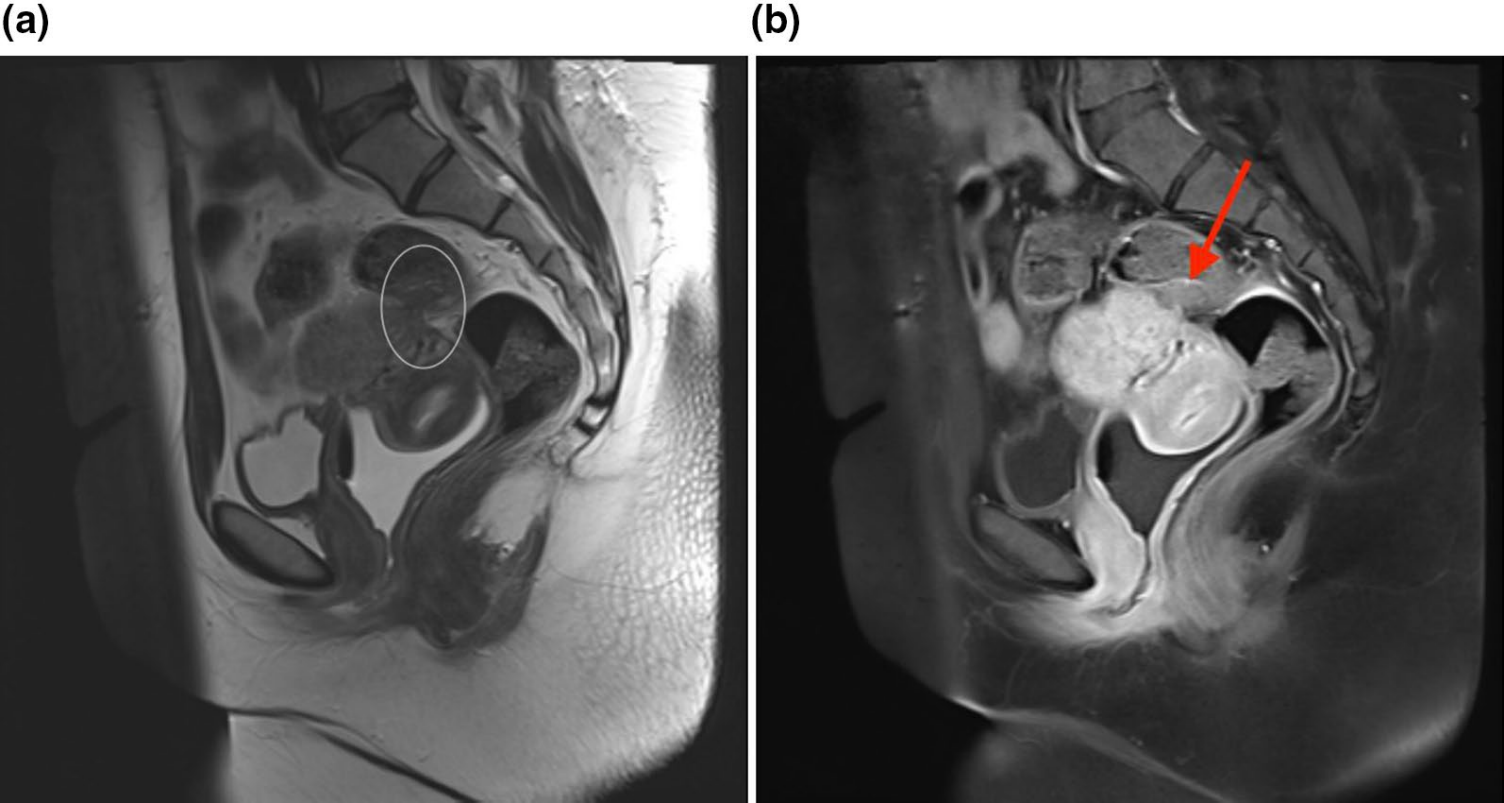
a T2 weighted sagittal MRI image showed involvement of retrocervical region as a site of DIE with some adhesions in the posterior cul-de-sac and rectal wall implant. b T1 weighted sagittal fat-suppressed image with contrast in the same patient showed rectal wall implant clearly (arrow)so size and depth can be easily measured for pre-operation planning
